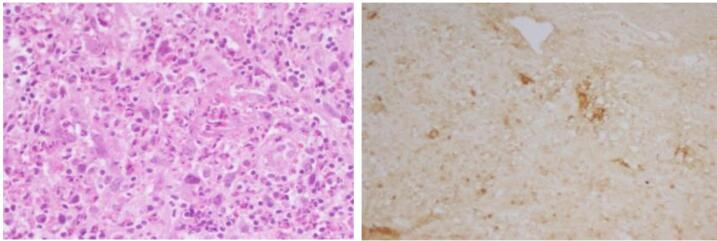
Chest radiography showed no mediastinal enlargement. Cervical, thoracic, abdominal, and pelvic scans showed multiple celiac, mesenteric, peri-duodenal, lumbo-aortic, and right primitive iliac adenopathy, gastric thickening, and moderate splenomegaly measuring 15 cm long axis (Figure 3).
